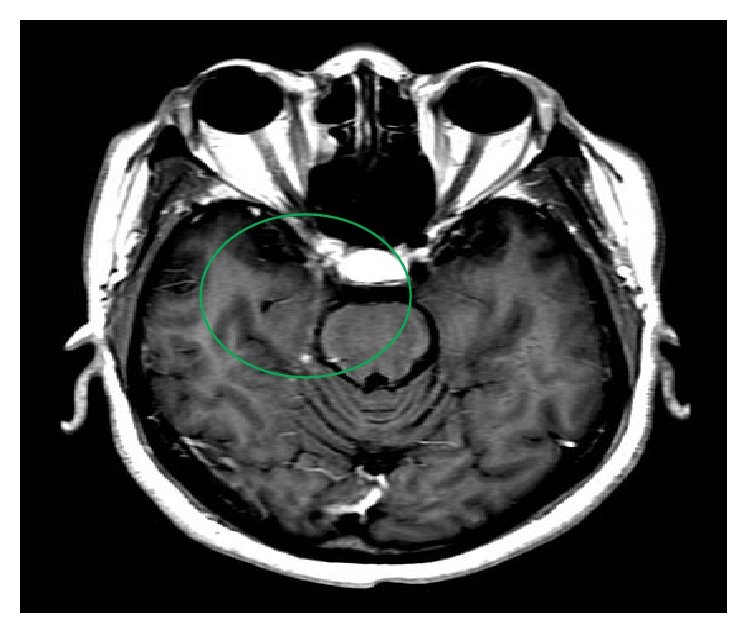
Subsequent cranial MRI showed resolution of the abnormal enhancement in the cavernous sinus area seen on the initial cranial MRI.
